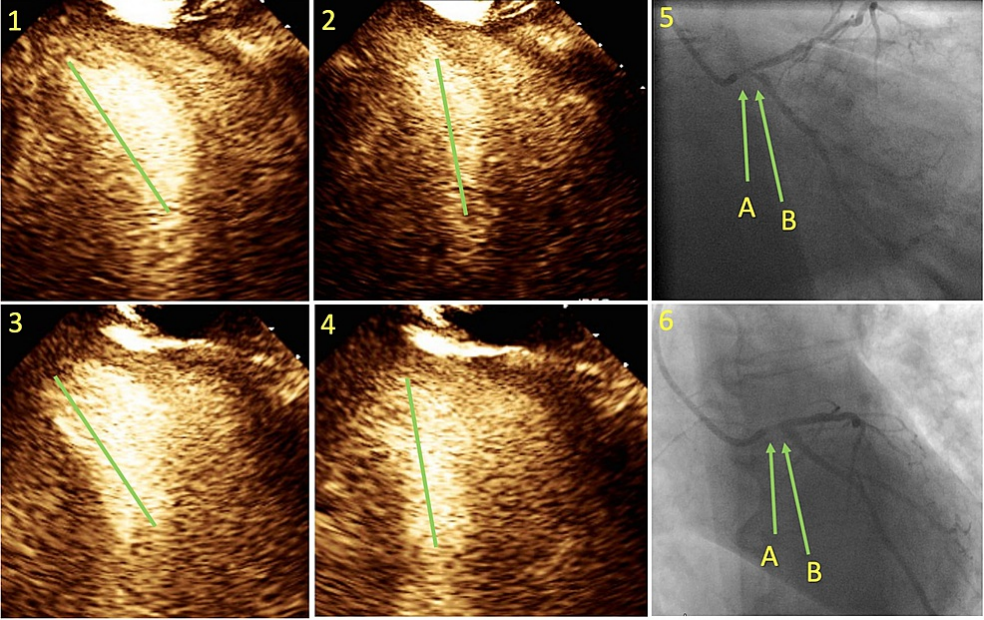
Dobutamine stress echocardiography and coronary angiogram images. Images 1 and 2 are two-chamber views of the heart in diastole and systole exhibiting tilt of the cardiac axis. Images 3 and 4 similarly represent the heart in four-chamber views. The green vertical lines in images 1, 2, 3, and 4 represent the imaginary line that passes through the center of the left ventricular cavity, i.e., the cardiac axis. Images 5A and 6A show severe left main stenosis with pressure dampening without contrast reflux. Images 5B and 6B show severely stenotic ostium of the left circumflex artery.
